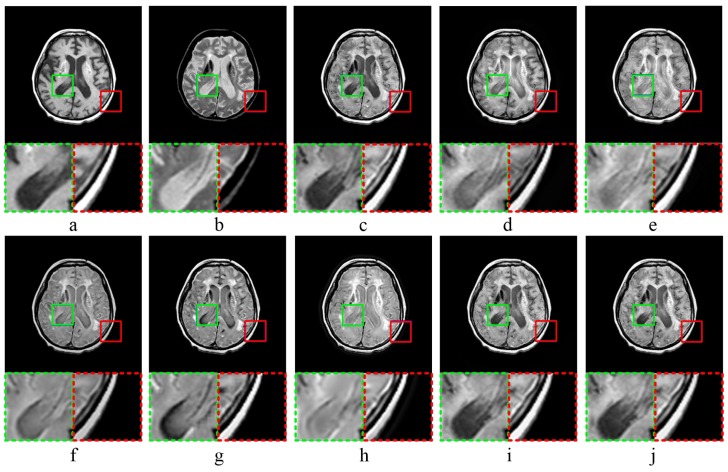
MR-T1 CMR-T2 image fusion experiments: (a,b) source images; and (c–j) the fused image obtained by MST-SR, NSCT-PC, NSST-PCNN, ASR, CT, KIM, CNN-LIU, and the proposed method, respectively. Two partially enlarged images marked in green and red dashed frames correspond to the regions surrounded by green and red frames in the fused image.
